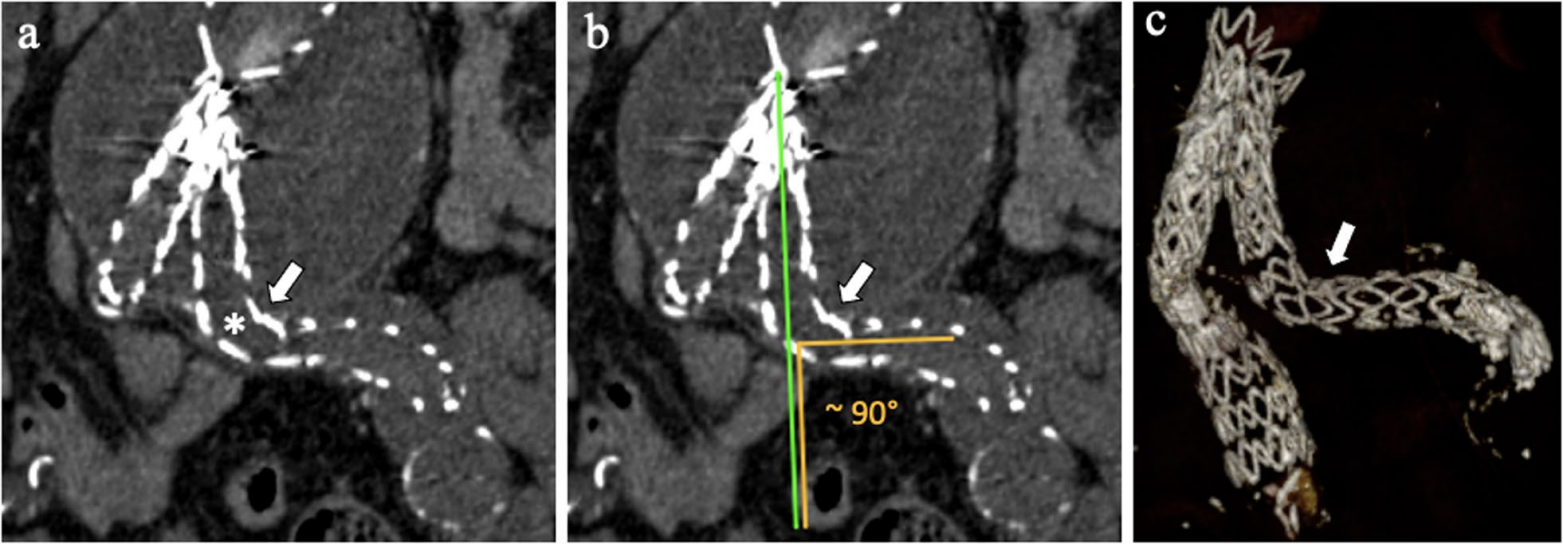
87-year-old man with left stent-graft-limb kinking. a, b Coronal CT images and (c) volume rendering reconstruction CT angiography image show left limb kinking (arrows) with angulation of about 90°, which is associated with endograft thrombosis (*)
